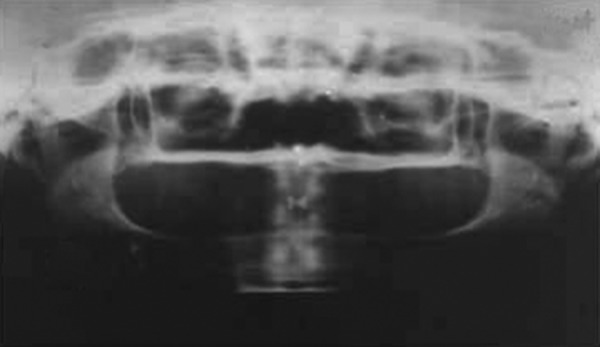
Radiograph showing complete anodontia
